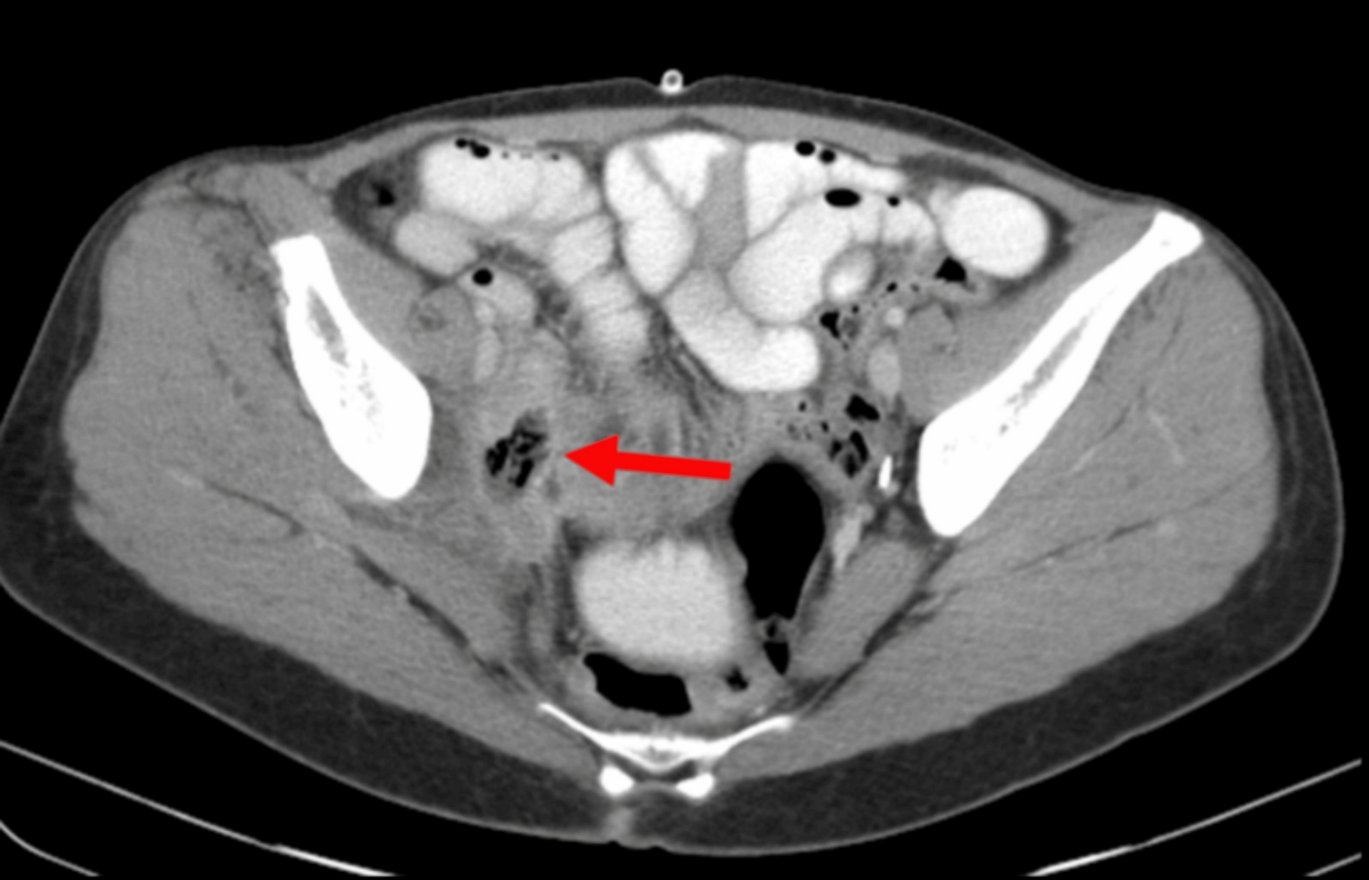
Pelvic collection of gas and fluid. The patient underwent CT imaging of the abdomen and pelvis with oral contrast, which demonstrated a gas and fluid collection along the right pelvic sidewall (arrow) measuring 2 × 3 × 2 cm, immediately adjacent to loops of small bowel and colon and associated with the previously noted abnormality.
